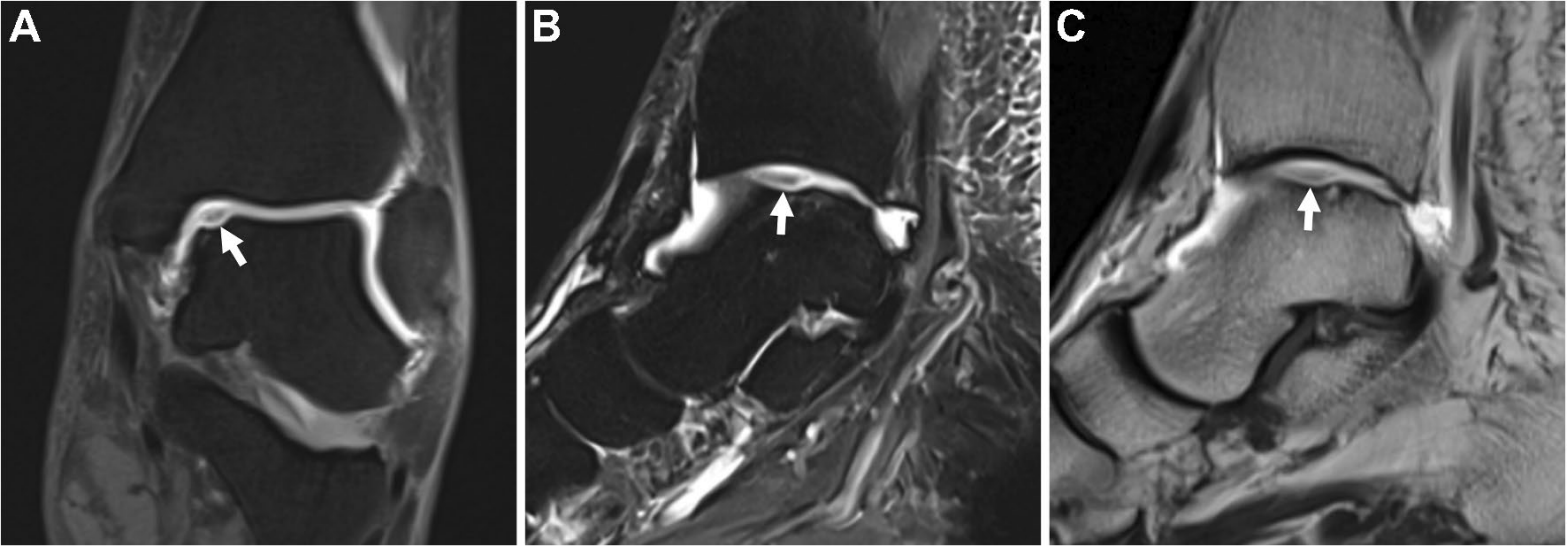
Osteochondral lesion. Sixty-two-year-old woman with ankle pain. Coronal T1-weighted fat-suppressed (A), sagittal STIR (B), and sagittal T1-weighted 3 T MR arthrogram images demonstrate an osteochondral lesion at the medial aspect of the talar dome with intra-articular contrast that insinuates into the interface with the talus (arrows), consistent with instability
